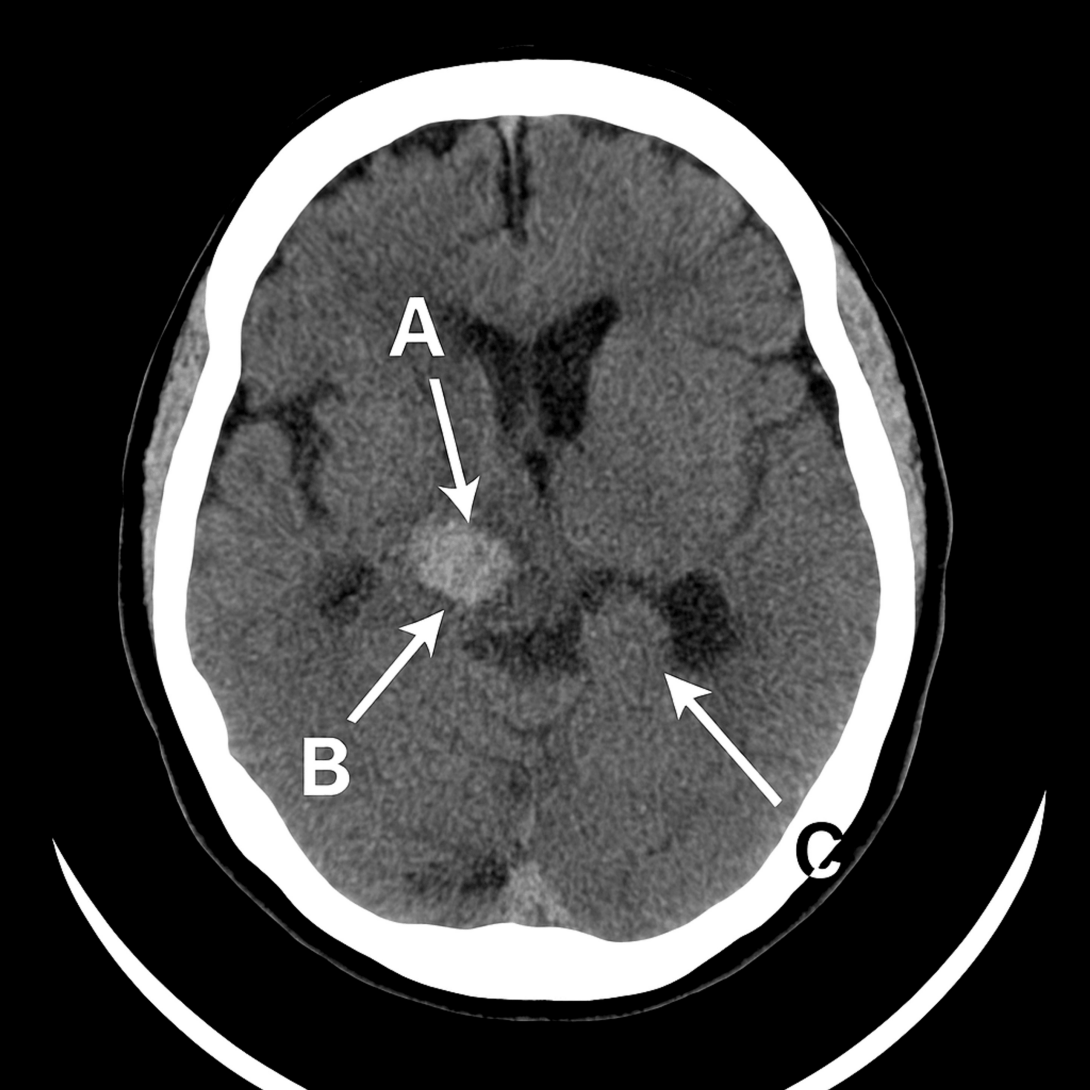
Axial non-contrast CT image of the brain demonstrating a stable acute hematoma (arrow A) in the left caudate nucleus, with intraventricular extension (arrow B). There is also mild prominence of the temporal horns (arrow C), consistent with communicating hydrocephalus. No evidence of infarction, trans-ependymal edema, or further hemorrhage is noted. A mild local mass effect is present.
